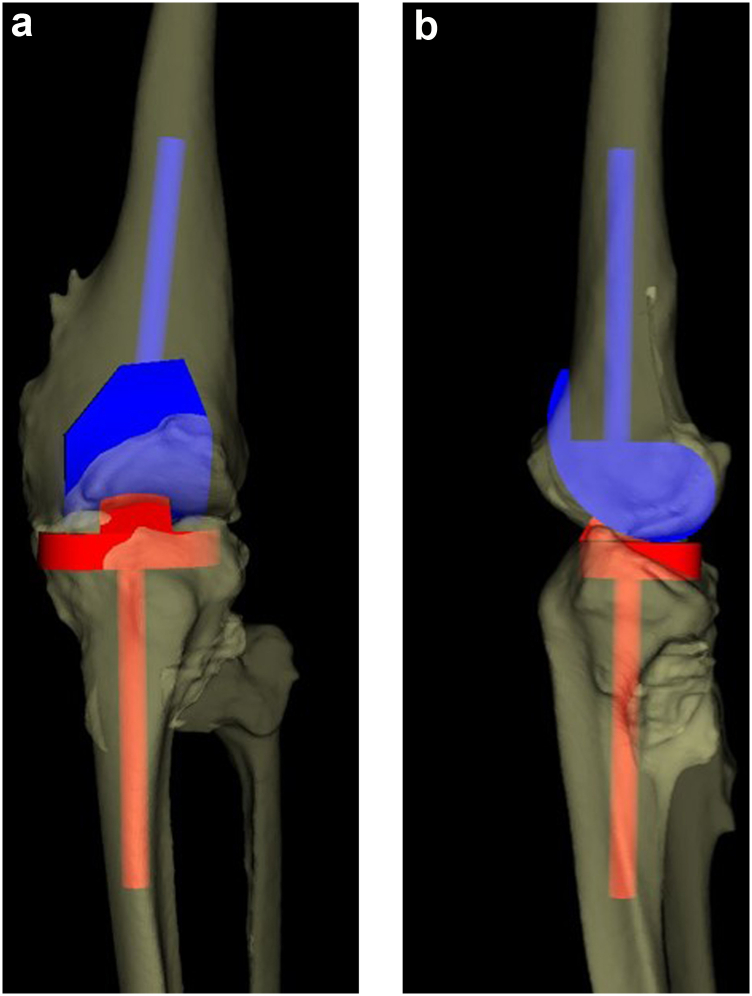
(a) Anteroposterior and (b) lateral computed tomography–based three-dimensional planning of total knee arthroplasty. We planned to use titanium-constrained hinge design prostheses (Kyocera Modular Limb Salvage system [KMLS system]; Kyocera, Kyoto, Japan).
